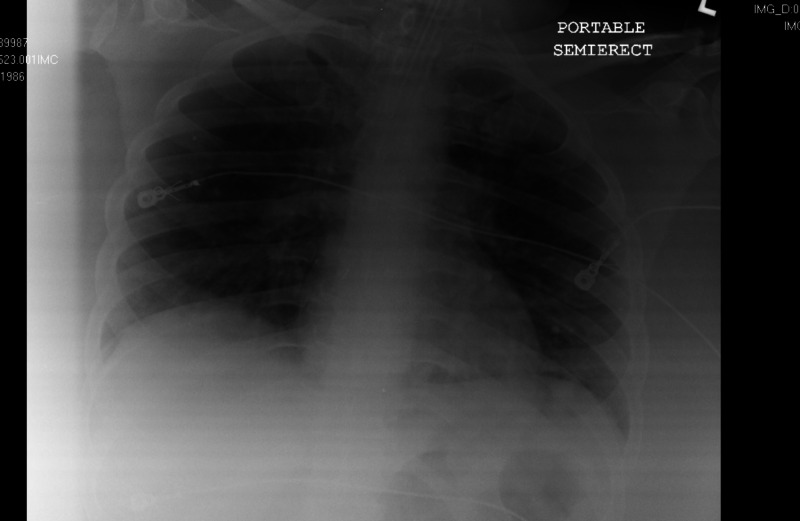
Case 1 chest X-ray on admission April 11, 2020
